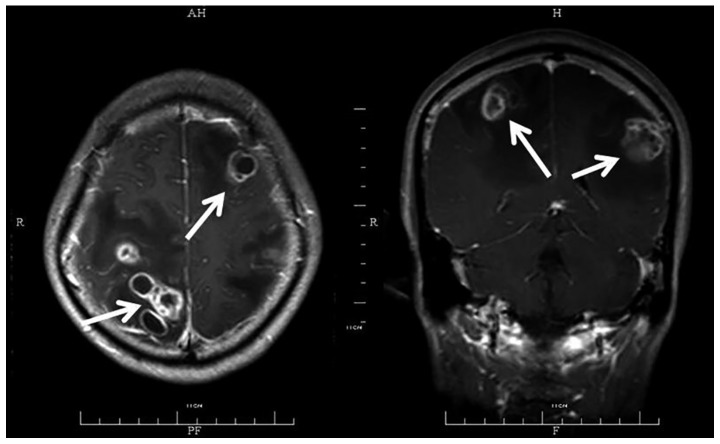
Contrast-enhanced magnetic resonance imaging scan of a 45-year-old male without any underlying diseases but with disseminated central nervous system nocardiosis, showing bilateral involvement of the brain. Multiple ring lesions with surrounding edema are shown (arrow), indicating multiple abscesses.
